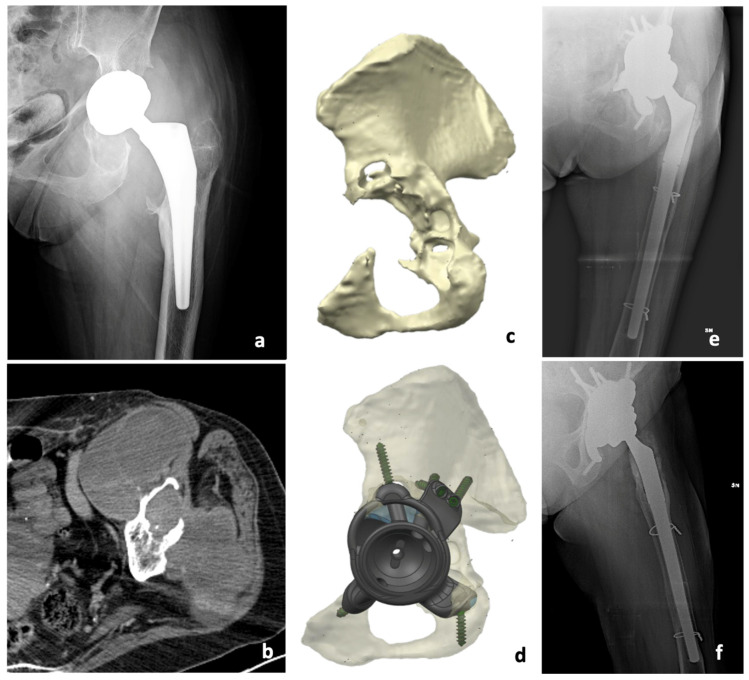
Imaging studies and surgical steps from a patient with pseudotumor of the hip treated with a two-stage procedure using an acetabulum custom made 3D printed. Preoperative X-ray (a) and CT scan (b) showing pseudotumor, as well as 3D CT scan performed after the first surgery (c) and 3D-printed model of the acetabular (d) with postoperative X-rays (e,f).
